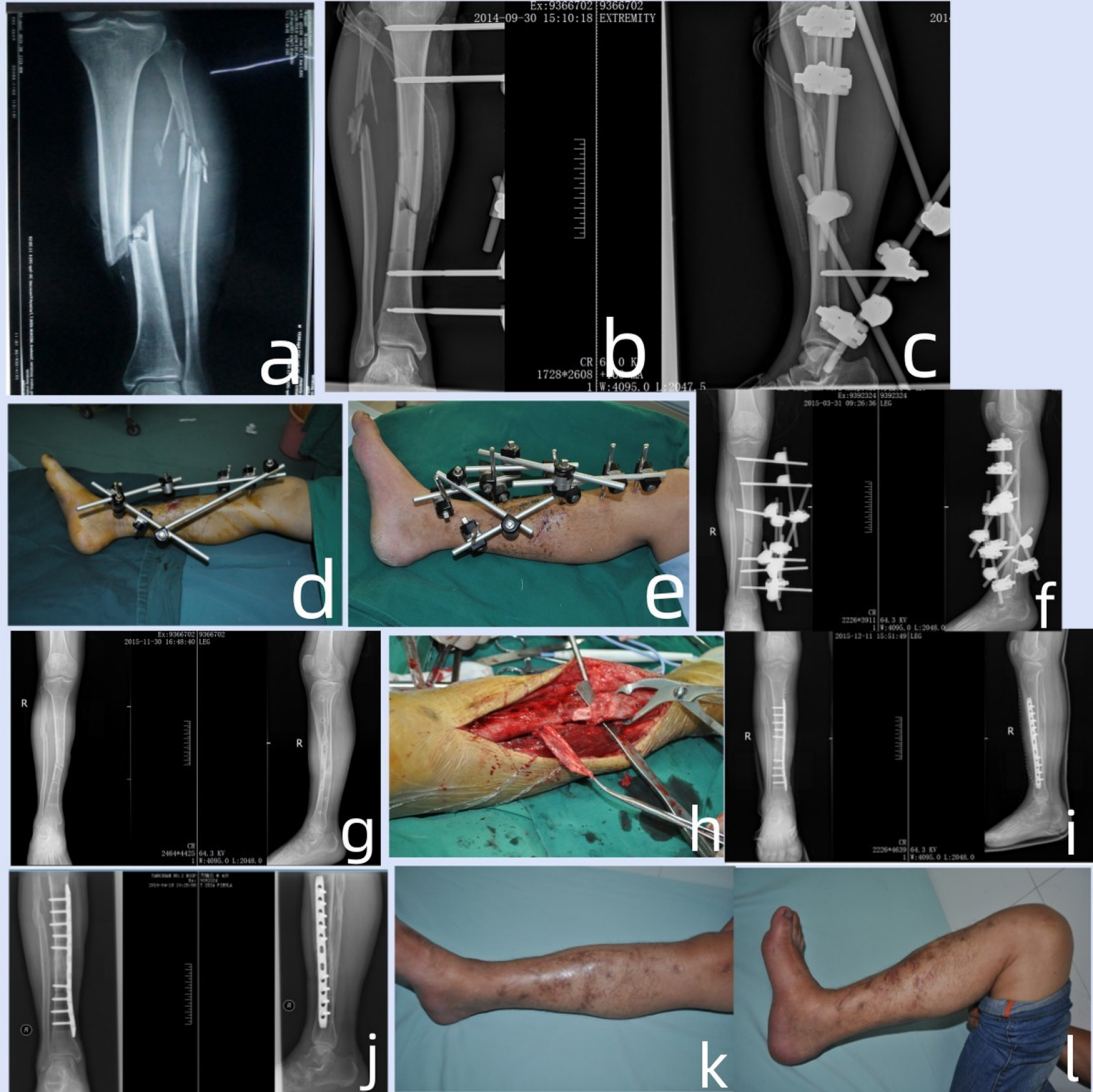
(a) The anteroposterior radiograph showing a Gustilo type IIIB injury with tibial and fibula bone fracture. (b) and (c) The lateral and anteroposterior radiograph after 2 weeks of the external fixator fixation of the affected lower extremity. (d) Appearance of the medial view of the wound after 2 weeks of the external fixator fixation. (e) The wound covered with flap after 2 weeks of the external fixator fixation. (f) and (g) The radiograph of injury after 6 months. (h) The operation of the transposition of the periosteal flap. (i) The radiograph after the internal fixation of tibial fracture with bone graft and periosteal flap transposition. (j) the radiograph showing the bone union after 4 months of the internal fixation of tibial fracture with bone graft and periosteal flap transposition. (k) and (l) The lateral and anterior view after 12 months of injury.
